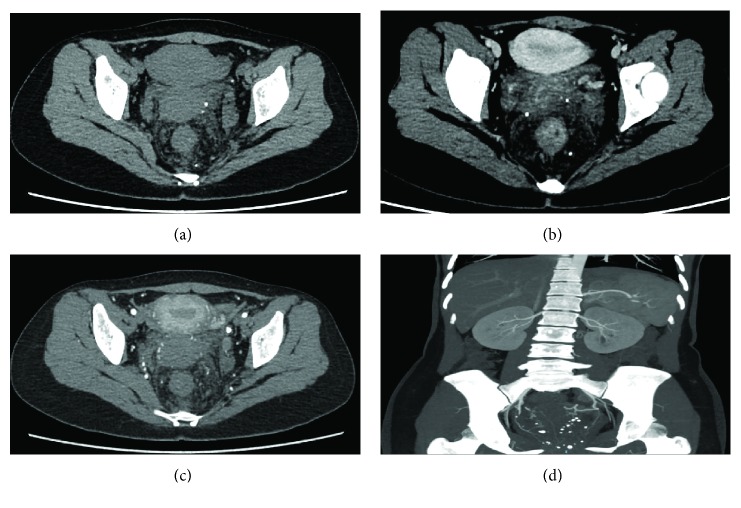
CT findings of DCHR. (a) Thickened rectal wall. (b) Enlarged serpentine vessels in the rectal wall. (c) Multiple calcification. (d) Serpentine vessels and multiple calcification.
